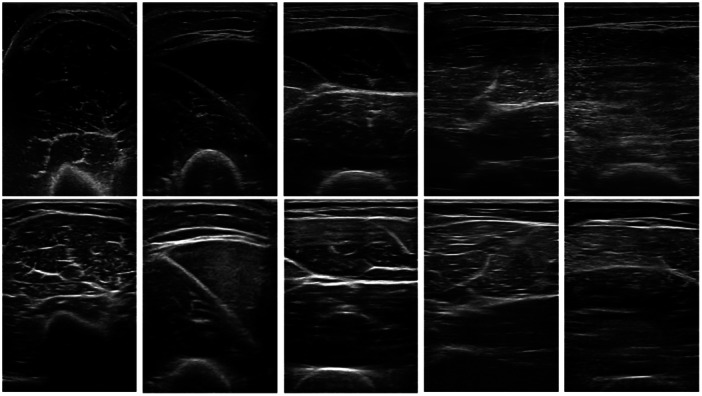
Ultrasound images acquired by the Vivid S5 and Lumify. The top row are images acquired by the Vivid S5, and the bottom row are images acquired by the Lumify. Sites of measurement from Left to Right for both rows: anterior upper arm, posterior upper arm, anterior thigh, posterior thigh, and posterior lower leg.
